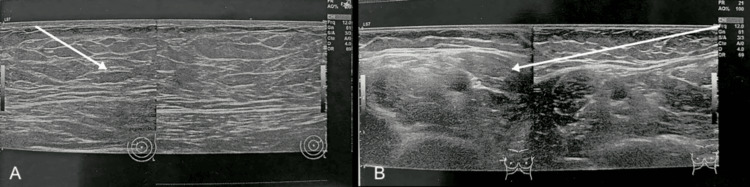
(A-B) Bilateral breast ultrasonography. (A-B) Arrows indicate retroareolar homogeneous glandular hypertrophy without focal lesions.
